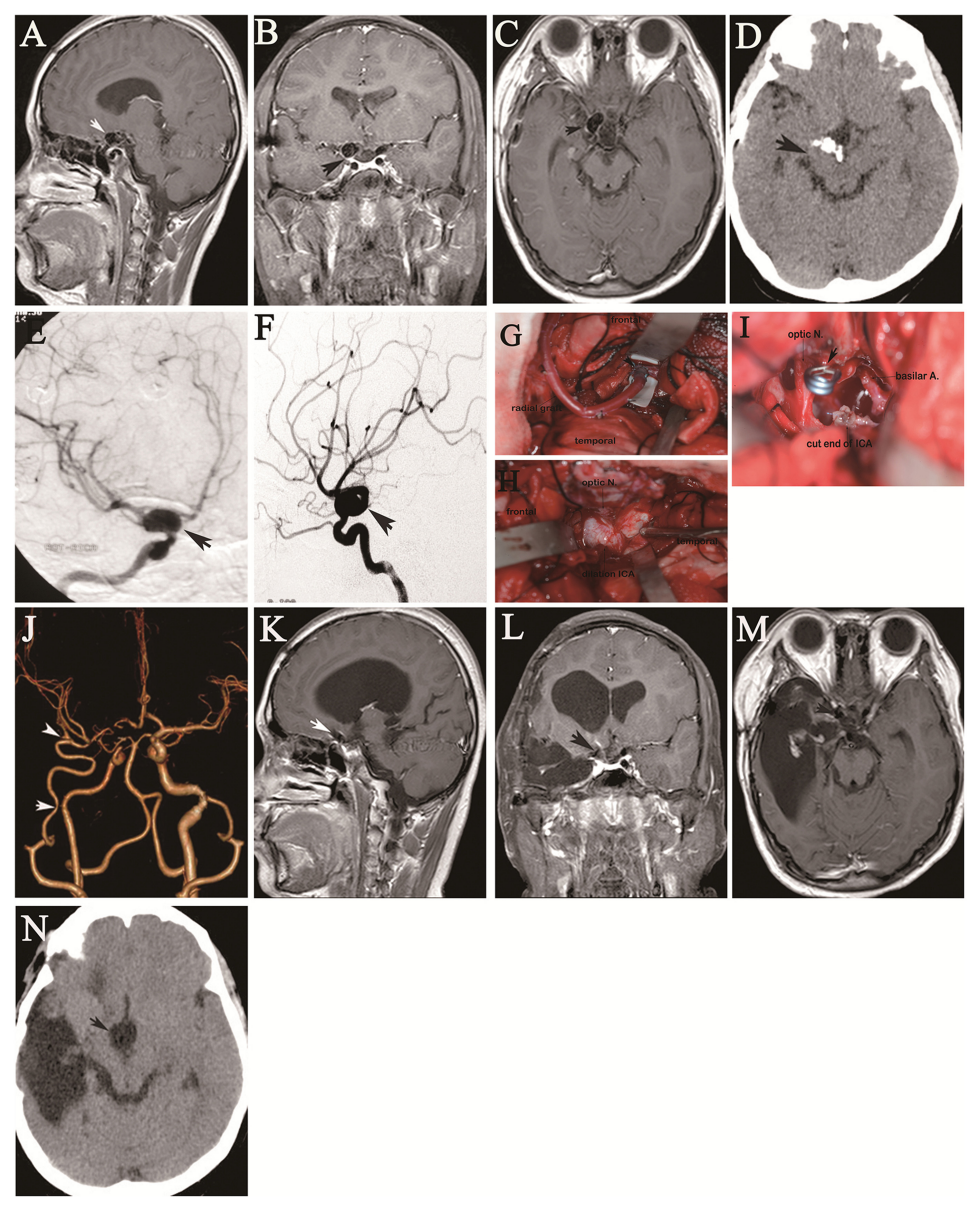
A 13-year-old girl was referred to our hospital with a 3-month history of severe headache and vomiting. Her neurological function was intact on admission. More than 5 years (September 2009) before she was referred to our department, she had undergone gross total resection of a craniopharyngioma at another institution. Preoperative sagittal (A), coronal (B), and axial (C) preoperative contrast-enhanced MRI shows the tumor in the intrasellar region complicated by fusiform ICA dilation (arrow). Preoperative axial CT (D) shows irregular tumor calcification. Preoperative right oblique (E) and lateral (F) DSA confirms dilation of the supraclinoid ICA with a maximum diameter of 21 mm (arrows). Intraoperative images show end-to-side anastomosis of the RA graft to the proximal M2 segment of the MCA *via* an opened Sylvian fissure through a frontotemporal craniotomy with resection of the zygomatic arch (arrow indicates the anastomotic site) (G). Image shows fusiform dilation lesion of the right supraclinoid ICA through exposure of the suprasellar region (arrow) (H). Image shows resection of the fusiform lesion, followed by clipping of the proximal and distal segments of the fusiform ICA dilation, and then resection of the tumor in the sellar region through the space left by resection of the fusiform ICA dilation (arrow) (I). Postoperative CT angiography showed patency of the IMA to the MCA and supraclinoid ICA sacrifice (the arrows indicate the anastomotic sites) (J). Follow-up sagittal (K), coronal (L), and axial (M) contrast-enhanced MRI at 3.5 years shows no tumor growth in the sellar region (arrow) and a right temporal pole defect related to evacuation of the postoperative hematoma. Axial CT demonstrates disappearance of tumor calcification (N).
